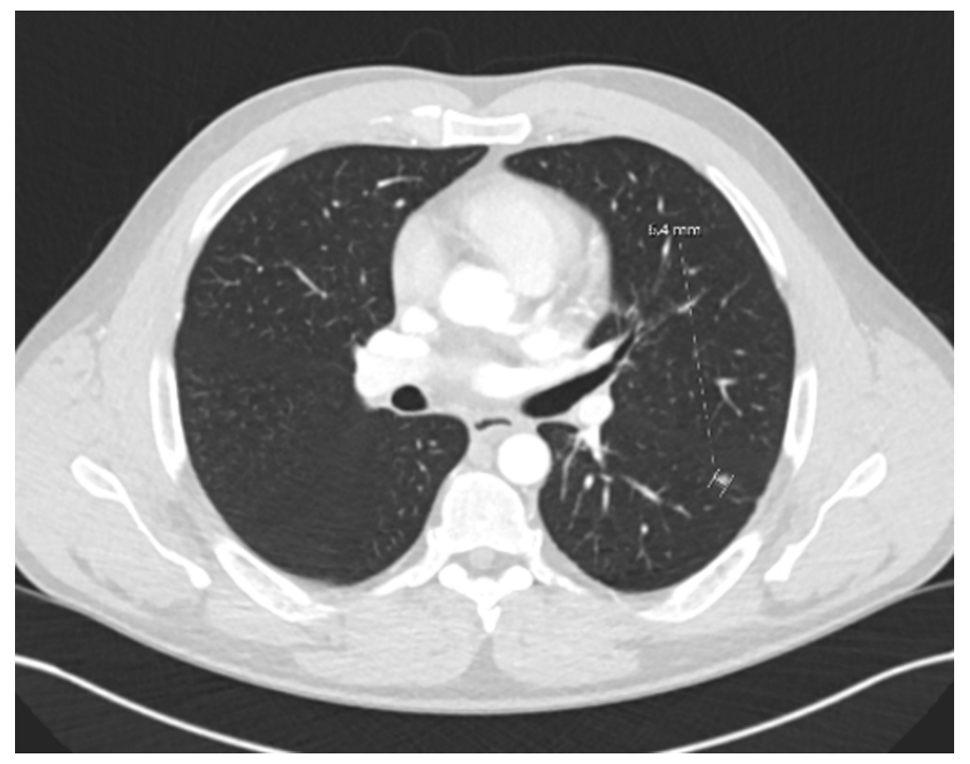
Axial contrast-enhanced computed tomography scan demonstrating a solitary pulmonary nodule in the left lower lobe, identified on routine surveillance in June, 2022.
